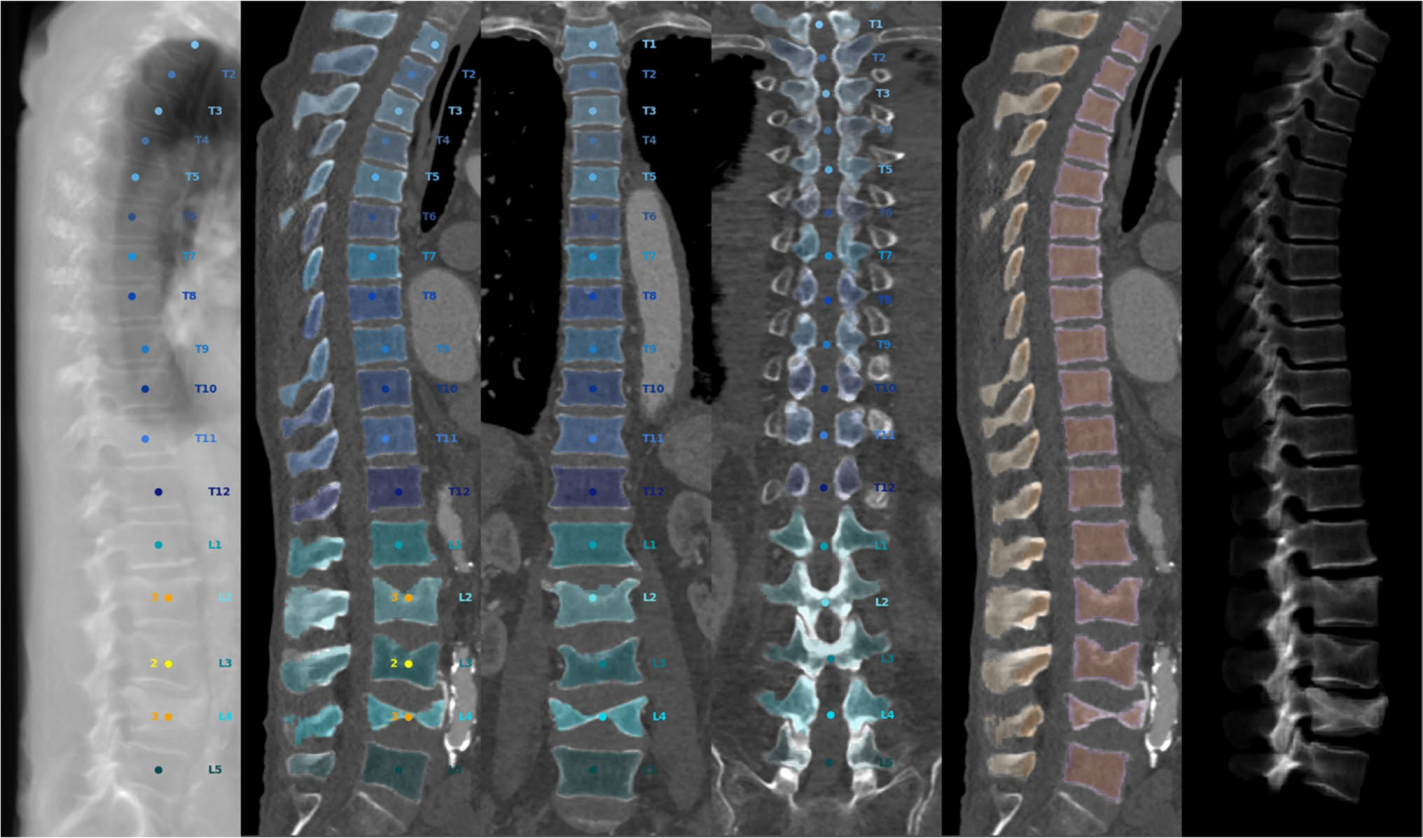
Automated spine labeling and segmentation of the thoraco-lumbar spine (T1-L5) in a routine TAVR planning CT using coronal and sagittal views, together with virtual radiographs in sagittal view. This patient had three lumbar vertebral fractures (L2-L4, Genant grades 2 and 3)
